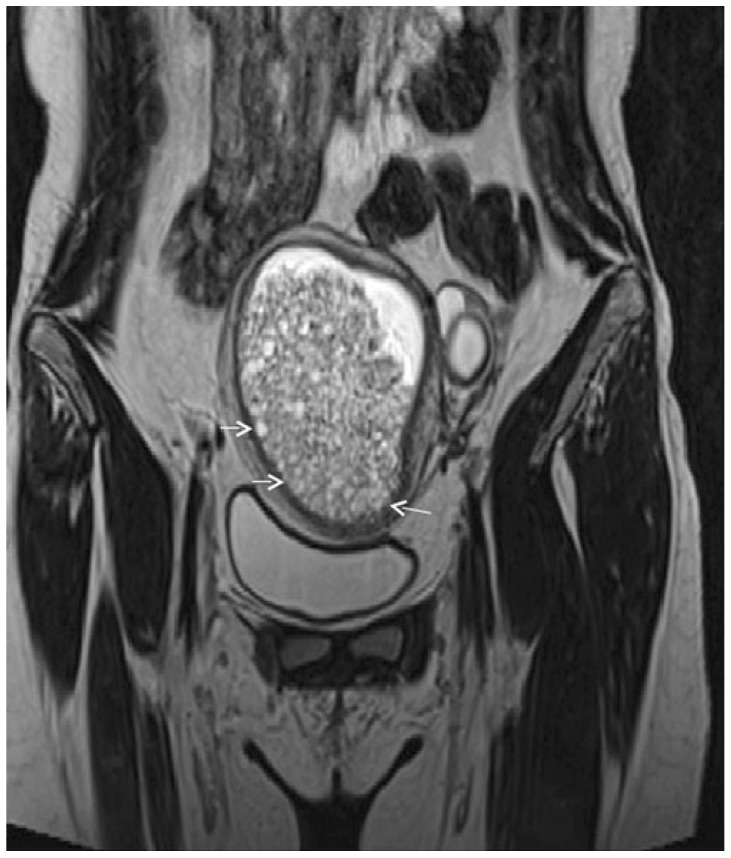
Coronal half-Fourier T2-weighted MR image in a patient with a hydatidiform mole. Coronal half-Fourier shows a large heterogeneous mass in the endometrial cavity; there is no evidence of myometrial invasion with the visualization of the normal dark myometrial line (arrows).
